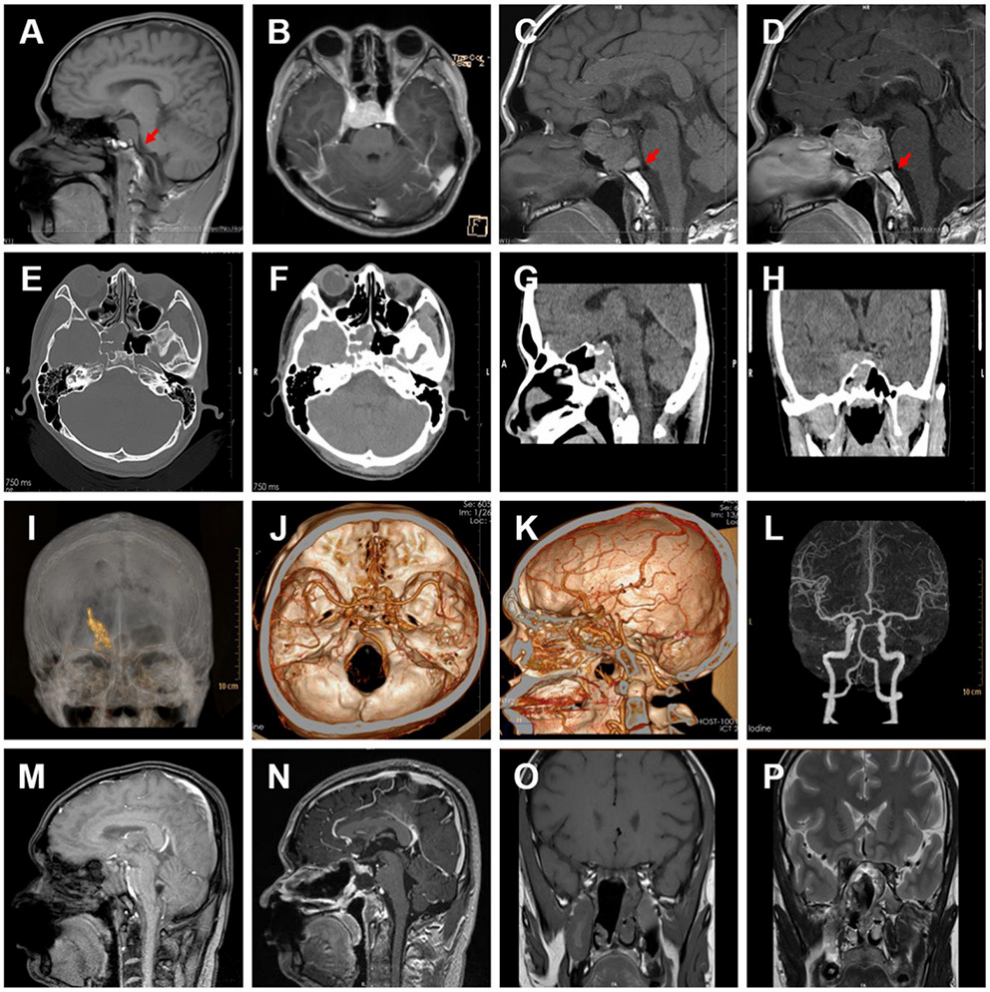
(A) MRI T1-weighted image from 2018-08-01, with the meningeal caudal sign in the red arrow. (B) MRI T1-weighted image from 2018-08-13. (C) MRI T1-weighted image from 2021-06-01. (D) MRI T1-weighted enhanced image from 2021-06-01, with the meningeal caudal sign in the red arrow. (E–H) CT images with the bone window. (I–K) 3D reconstruction of CT scan shows the tumor was posterior to the basilar artery, anterior to the hypophysial stalk, and surrounded by the internal carotid artery. (L) Pre-operative CTA contrast imaging. (M–P) Postoperative images.
